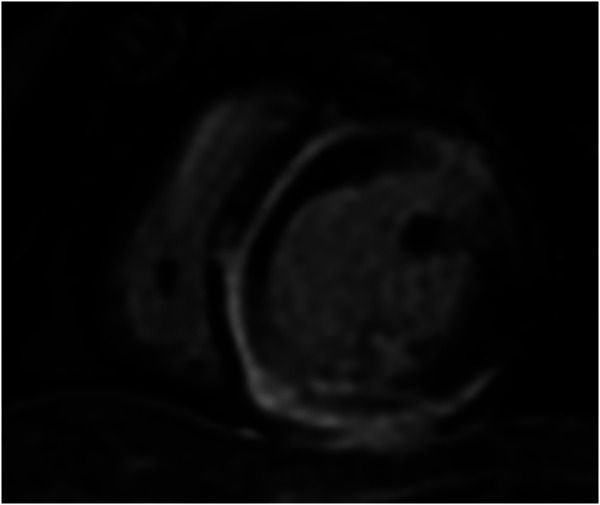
Serial cardiac magnetic resonance imaging demonstrating significant progression of disease with near circumferential subepicardial delayed enhancement of the left ventricular myocardium.
